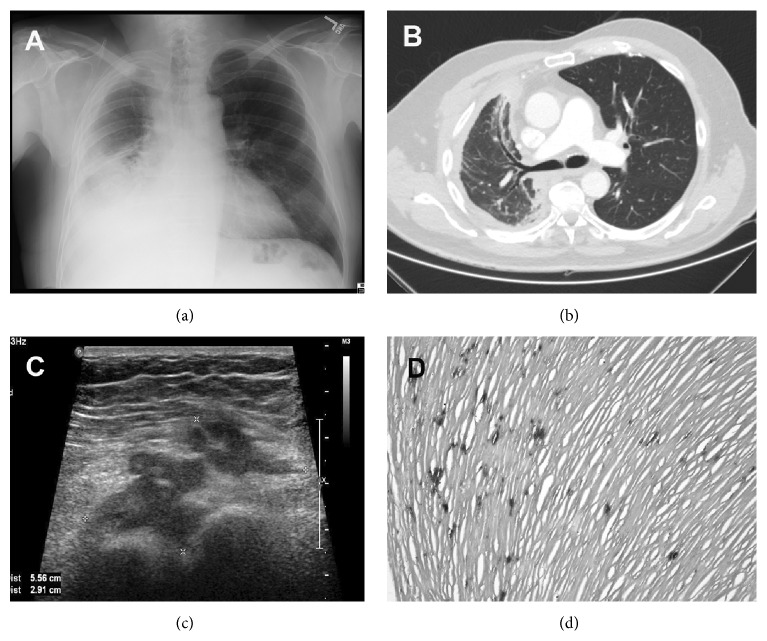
(a) Anteroposterior chest X-ray shows pleural thickening along the right lateral chest wall and blunting of the right costophrenic angle. (b) A chest CT scan shows extensive pleural thickening on the right side and calcified pleural plaque on the left side. (c) A breast ultrasound shows an irregular, hypoechoic mass measuring 5.6 × 2.9 × 3.6 cm. A portion of the mass involves pectoralis muscle and extends into the intercostal muscles. (d) Pleural biopsy shows plaque formation with dense fibrosis, minimal inflammation, and dystrophic calcification with no evidence of malignancy.
